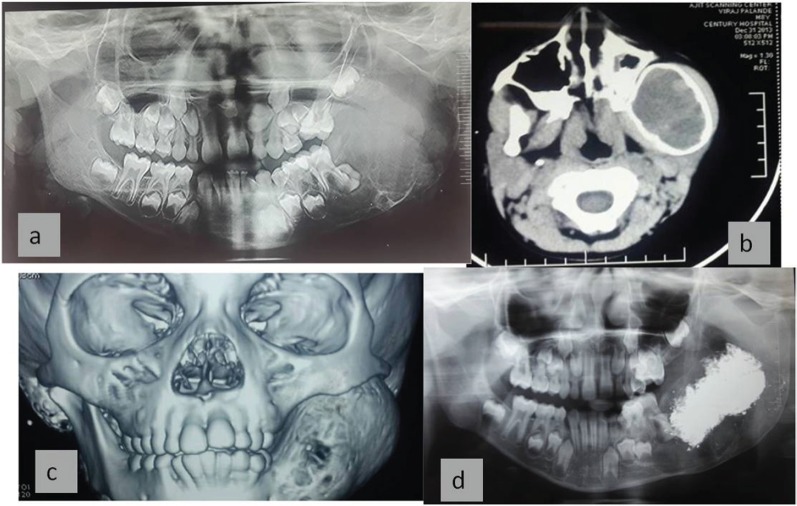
X-ray and CT scan show (a) large multilocular mixed radiolucent radiopaque lesion involving left side of mandible extending from mandibular, second deciduous molar. Superiorly it extends up to condylar and coronoid processes. Inferior margin showed multiple septae with thinning of the cortical plates, Anterior displacement of the tooth bud 37 was noted. (b) The CT sections show large severely expansile lesion in the left ramus of the mandible extending up to the cortical plates. (c) 3D CT shows expansion and thinning of cortical plates localised areas with perforation of cortical plates noted. (d) shows postoperative follow-up after two months with bony healing inferiorly.
